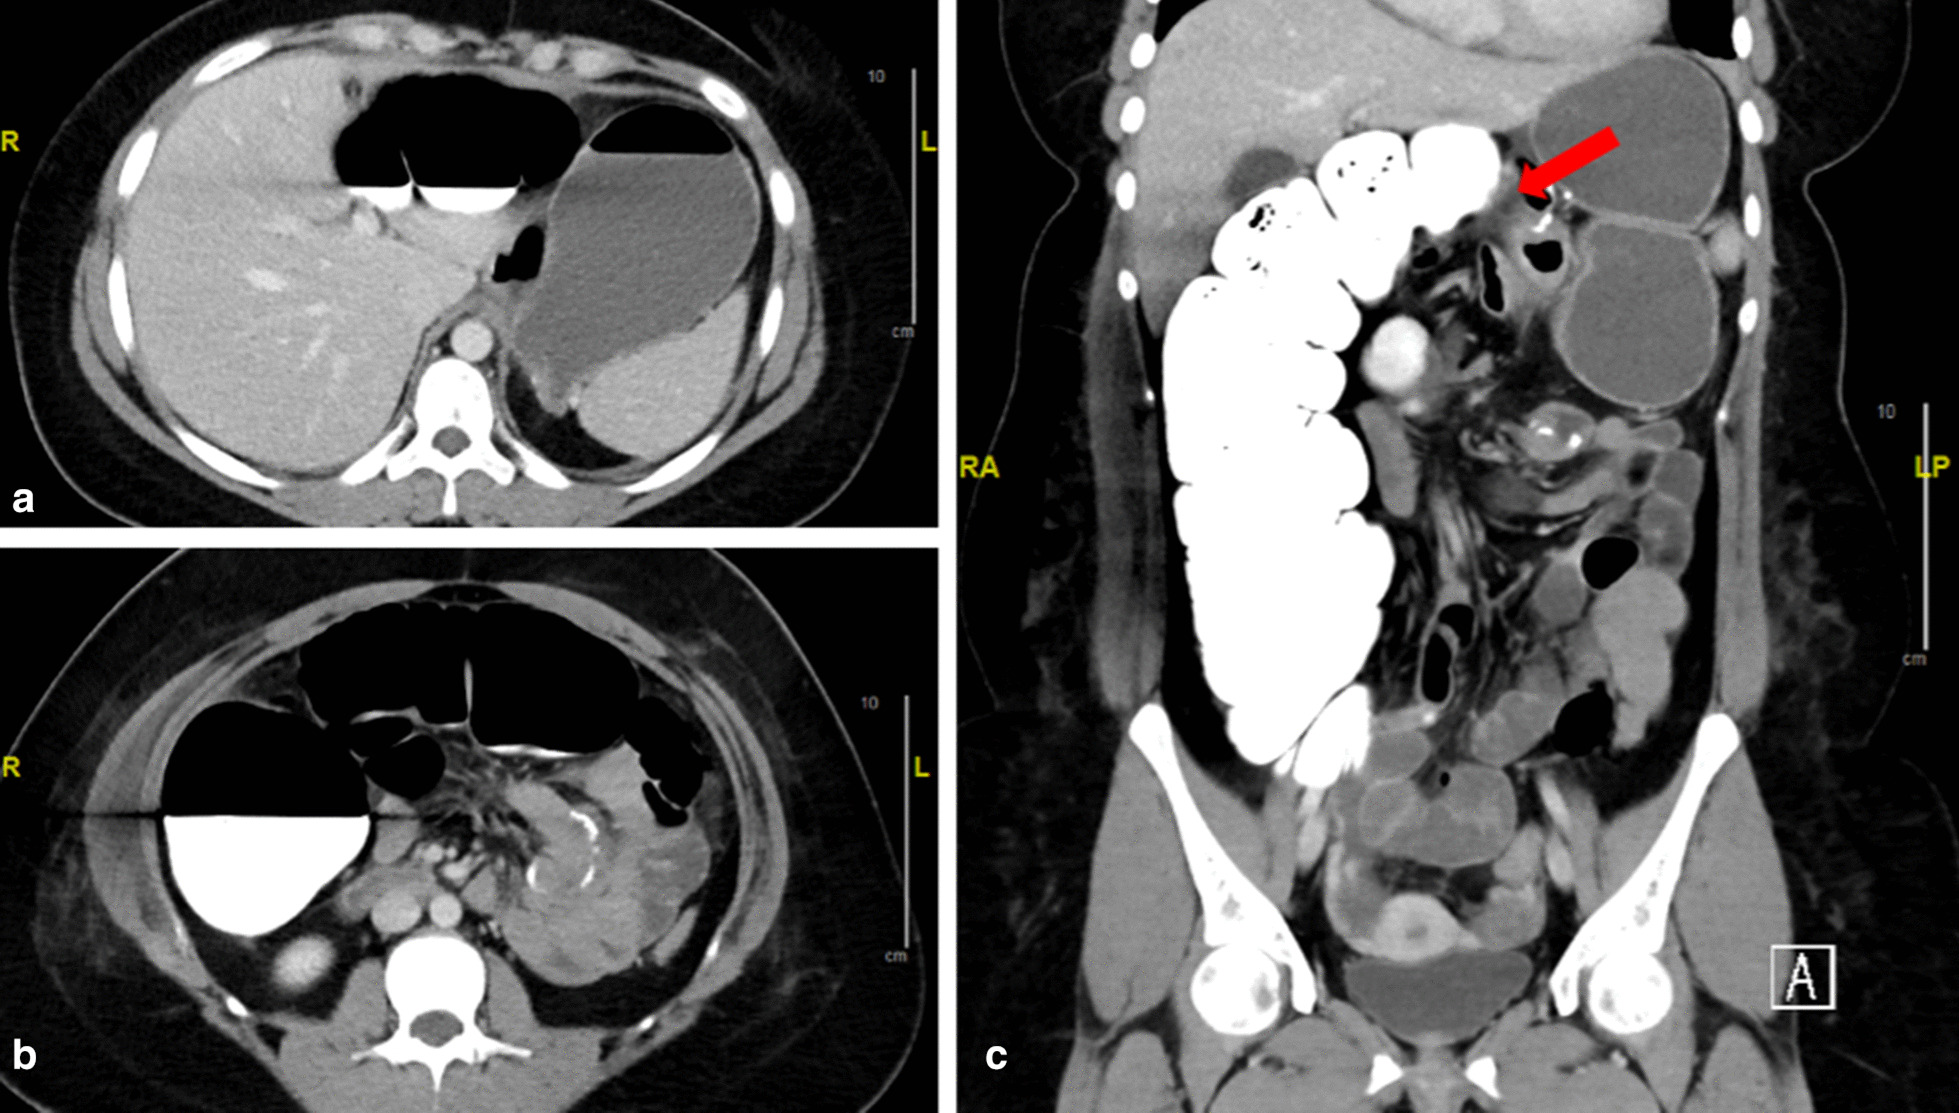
Abdominal computed tomography scan on the fourth postoperative day showing signs of colon obstruction. a Dilated and fluid-filled remnant stomach. b Dilated right hemicolon, normal presentation of gastro-jejunostomy. c Dilated right hemicolon with change of caliber, normal presentation of jejuno-jejunostomy. Red arrow points to the antecolic alimentary limb compressing transverse colon
